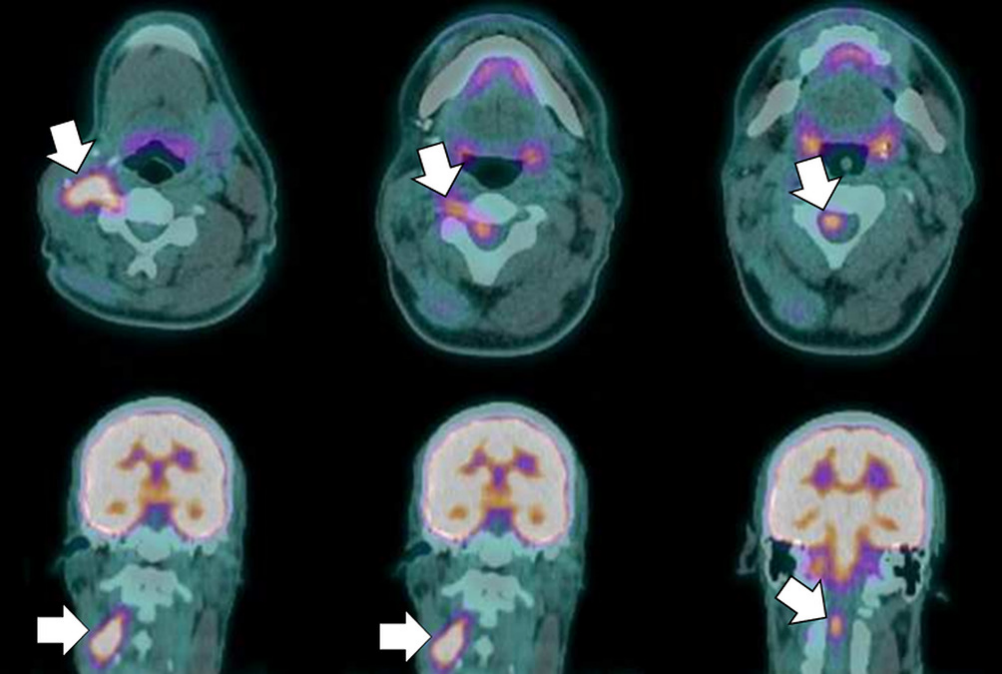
Positron emission tomography/CT axial and coronal images demonstrate an ^18^F-fludeoxyglucose-avid mass in the right side of the neck with tubular extensions along the C3 and C4 nerves into the spinal cord, indicating perineural spread (arrows).
